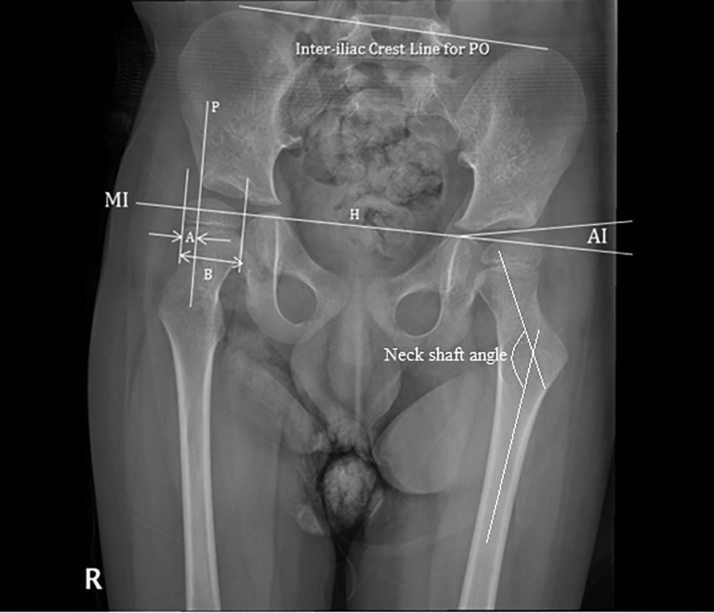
Hip radiography method for measuring MI, AI, neck-shaft angle, and PO.
